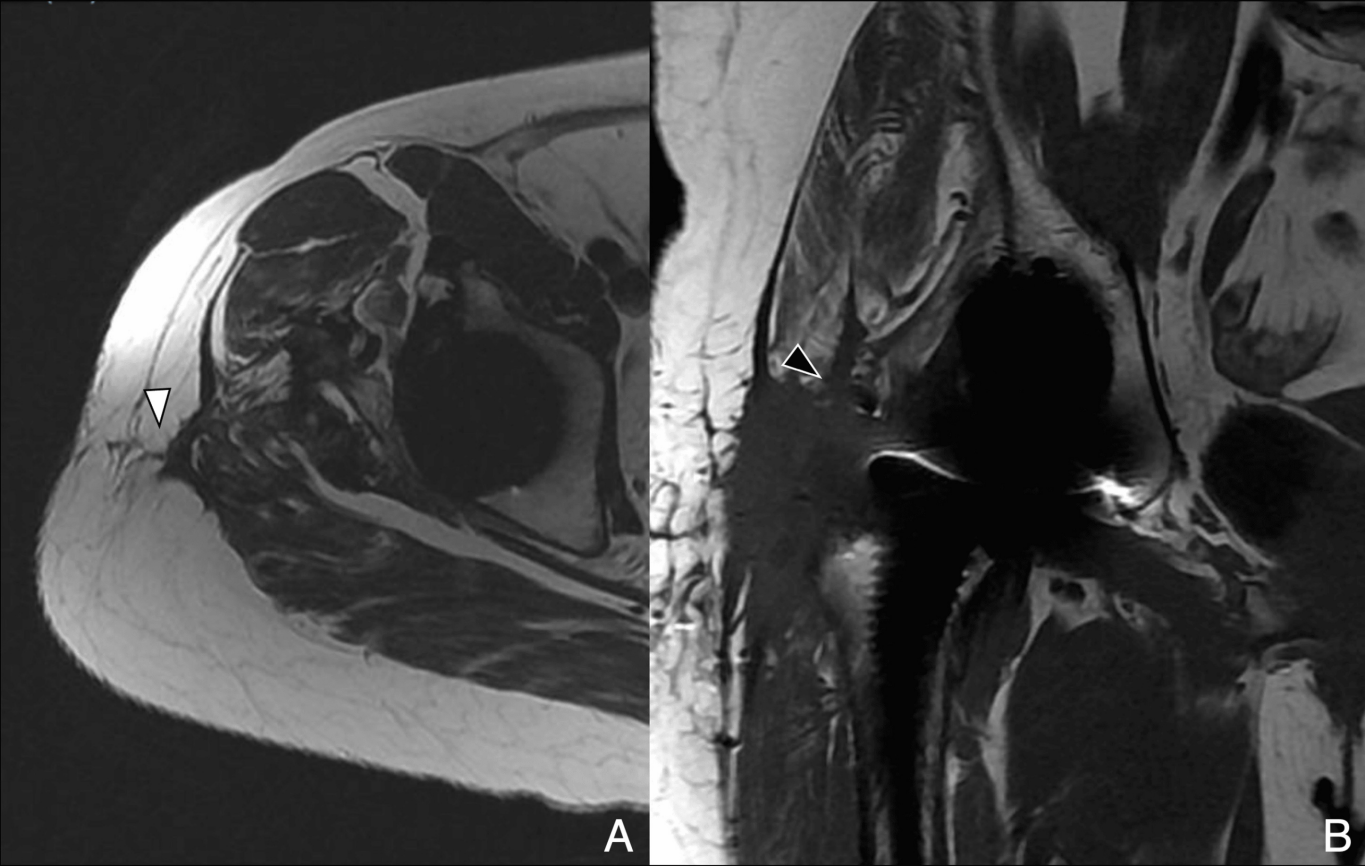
Lateral approach: postoperative MRI (A) Axial T2-weighted image shows subcutaneous scar tract (white arrowhead). (B) Coronal T1-weighted image shows a scar at the myotendinous junction of the gluteus minimus (black arrowhead) and scar along the gluteus medius dissection plane. Image Credit: Juan Pablo Muñoz, corresponding author
